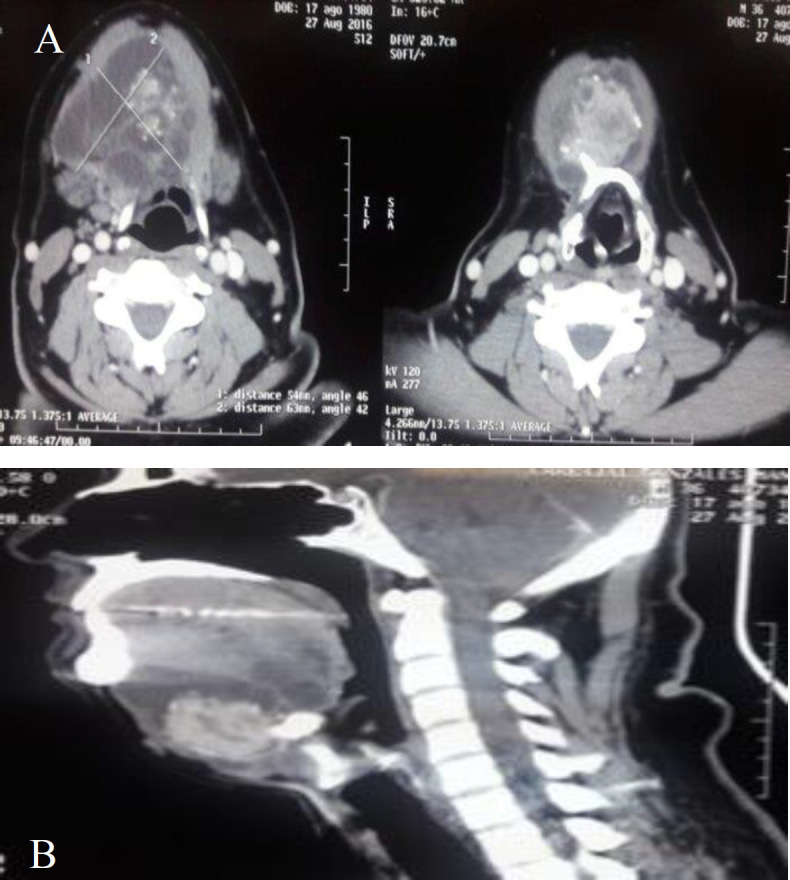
A. Contrast-enhanced computed tomography (CT) neck soft-tissue window axial view of patient that shows a mixed inframentonian heterogeneous tumor associated with small, mostly peripheral calcifications, that measured approximately 67x44x66 mm, conditioning compression of the aerodigestive tract B. Contrast-enhanced CT neck soft-tissue window sagital view of patient
